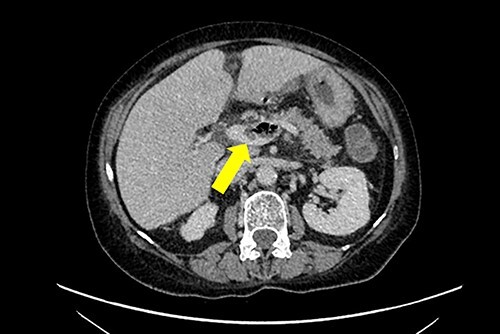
Axial section of a non-contrast CT scan with yellow arrow showing portal vein thrombosis with extension to the left intrahepatic portalvein.
